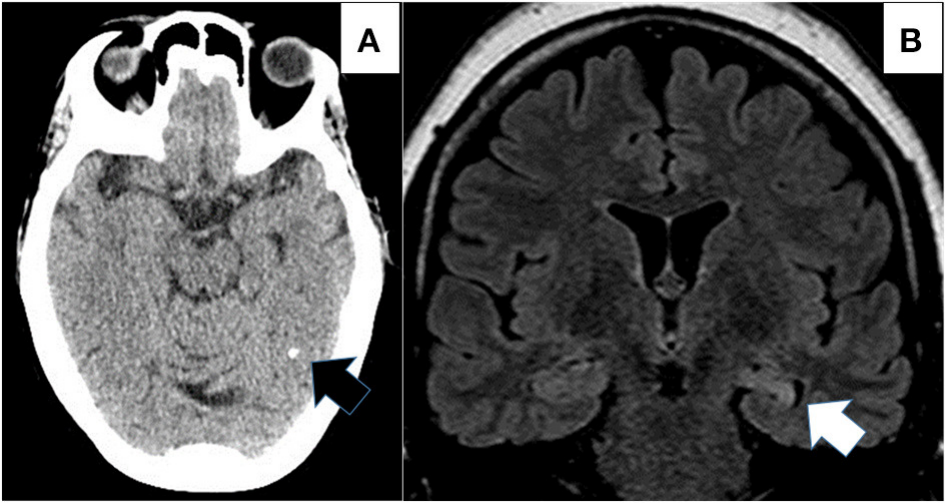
(A) CT-scan showing a single calcification (black arrow) highly suggestive of cNCC. (B) MRI FLAIR coronal imaging showing findings of hippocampal sclerosis (white arrow), typically observed in patients with mesial temporal lobe epilepsy associated with hippocampal sclerosis.
